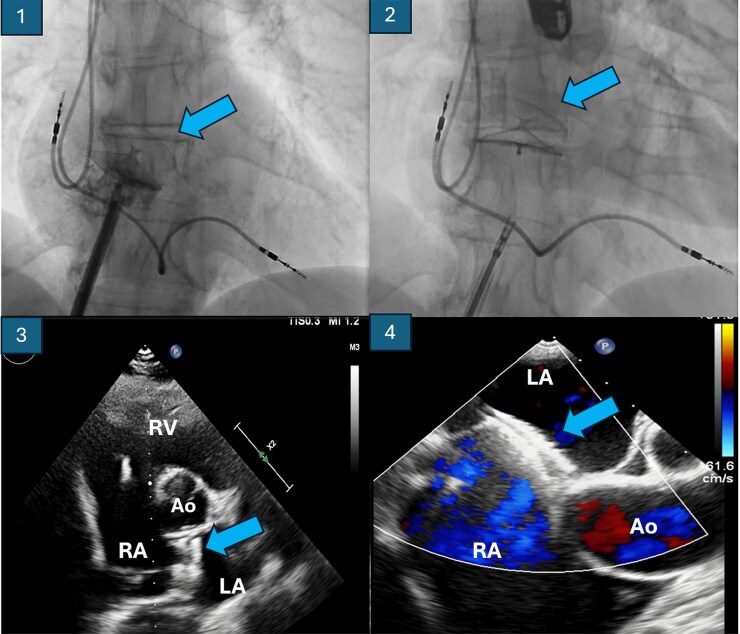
Closure device for foramen. In the images above (1 and 2), fluoroscopy shows the deployment of the device. In the images below, the outcome of the implant is visualized through transthoracic echocardiography on the left (3) and transoesophageal echocardiography (4), without evidence of residual shunt. Ao, aorta; LA, left atrium; RA, right atrium; RV, right ventricle.
